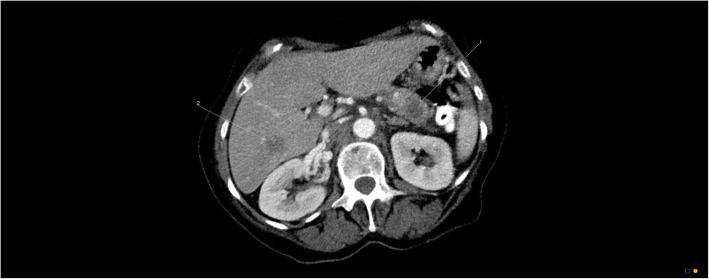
CT chest/abdomen/pelvis showed widespread metastases. Left arrow points to hepatic lesion, and right arrow demonstrates the pancreatic mass
